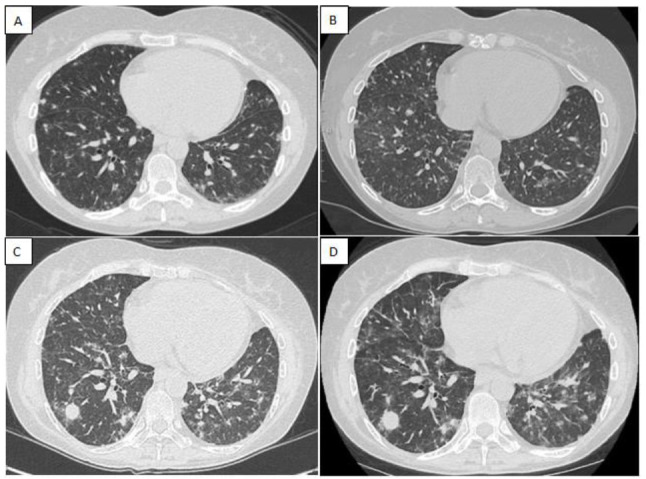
(A) Lung CT shows bilateral parenchymal nodules with predominantly peribronchovascular distribution with slightly blurred margins, surrounded by ground glass areas; (B) Slight increase in micro nodularities and ground glass findings; (C) Appearance of major parenchymal consolidations with air crescent sign in the lateral segment of the right lower lobe; (D) Dimensional increase of the previously described consolidation with simultaneous worsening of the remaining findings.
